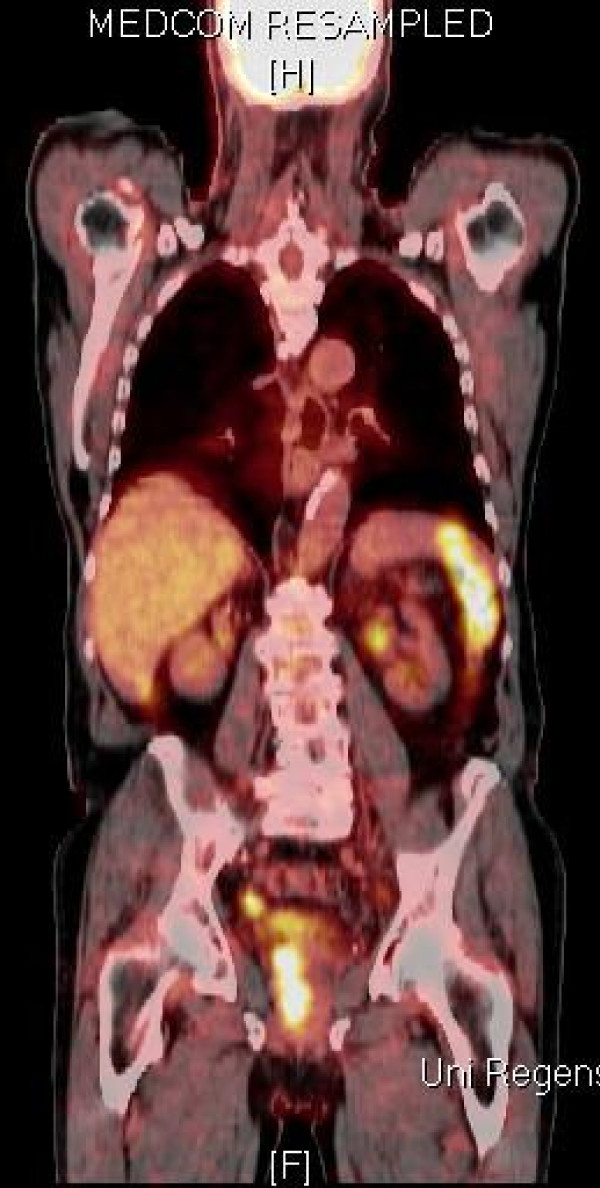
PET-CT scan, August 2008: declining accumulation in projection to the right seminal vesicle and the right parailiacal lymph nodes.
